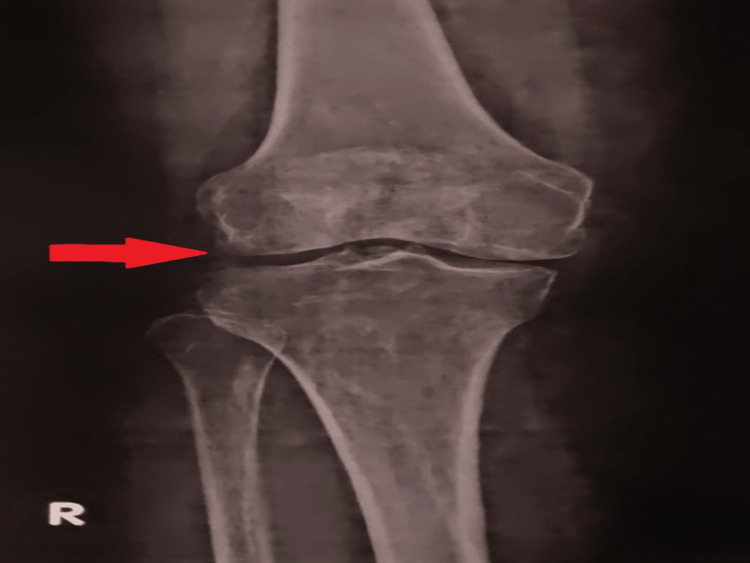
X-ray showing reduced joint space and osteophyte formation.
